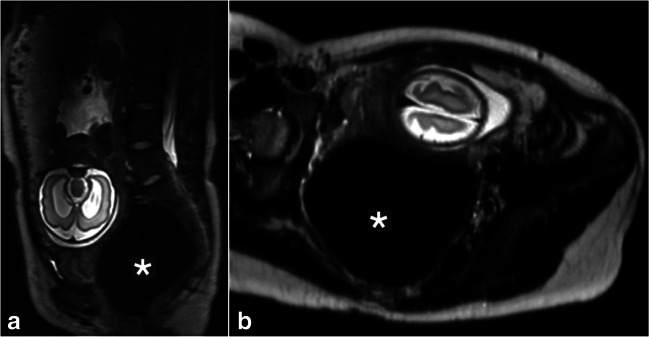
Grossly dilated rectum in a 21-year-old woman imaged at 29^+0^ weeks’ gestation due to bilateral fetal ventriculomegaly found on ultrasound. a, b Sagittal (a) and coronal (b) T2 turbo spin echo MR images show rhomboencephalosynapsis with severe ventriculomegaly secondary to aqueductal stenosis and a reduction in cortical folding. The images also show a level III maternal incidental finding of a grossly dilated rectum (*asterisk*)
